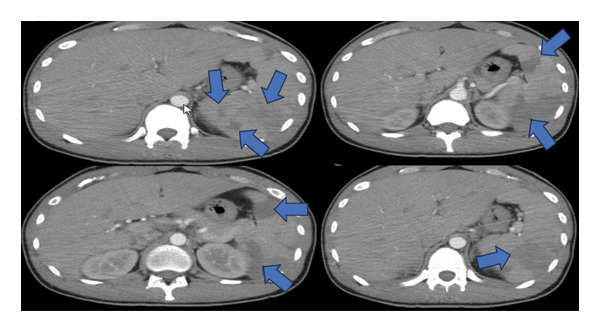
Still images from CT abdomen and pelvis from the patient’s 2^nd^ presentation to the referring hospital, 6 days after being discharged after his first presentation. These images demonstrate significantly enlarged splenic infarcts (blue arrows), splenomegaly, and hepatomegaly.
